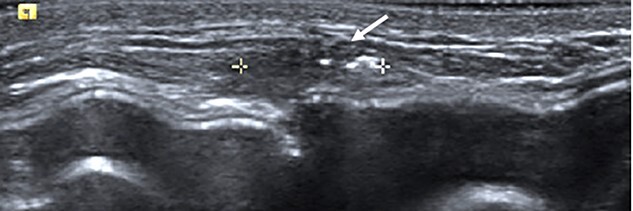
Ultrasound of the left central breast identified an irregular mass on the capsule of the implant with a focus of hyperechoic calcification (arrow).
